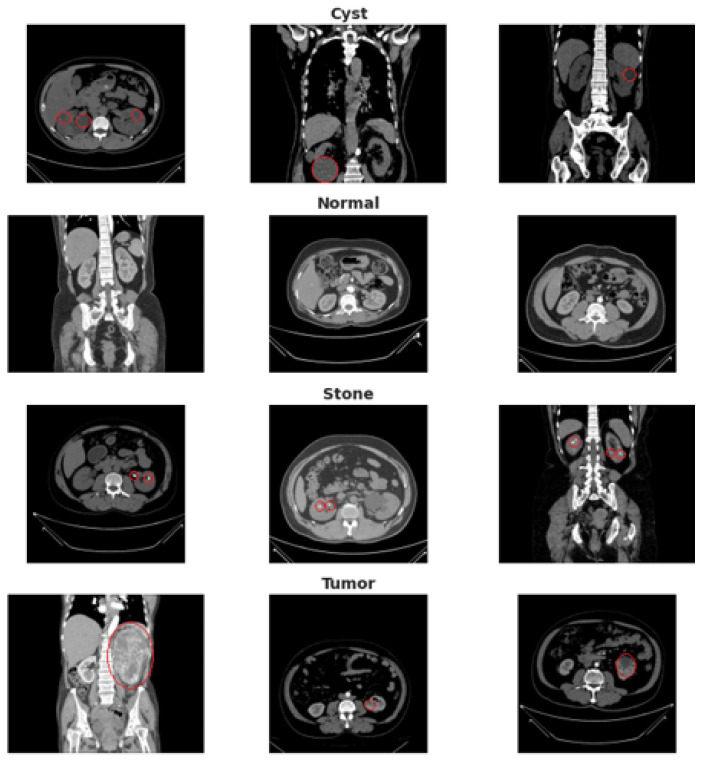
Dataset overview showing representative CT slices with corresponding annotations for Normal tissue, Cyst, Tumor, and Stone. The red circles highlight the region of interest (ROI) manually verified by the radiologist, indicating the precise anatomical location of the pathology in each example.
